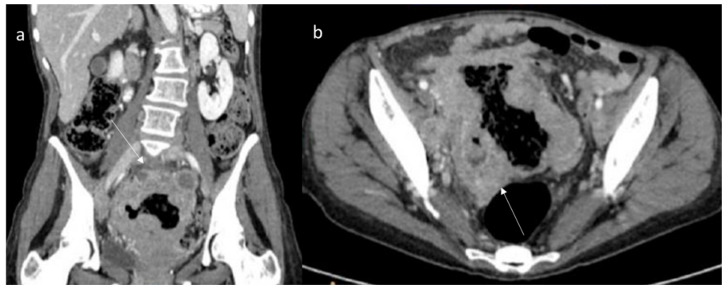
MPR coronal (a) and axial CT (b) assessment (portal phase of contrast study) of Sigmoid Lymphoma (arrow). The sigma has thickened walls with inhomogeneous contrast enhancement.
